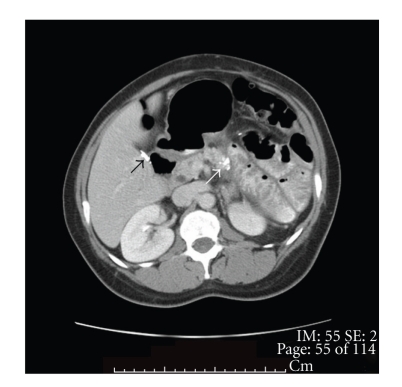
46-year-old woman with pancreatic cancer and prior distal pancreatectomy. CT scan with contrast through the pancreas. There is calcification at the cut surface of the pancreas (white arrow). No residual tumor is seen at this site. Cholecystectomy clips are present in the gall bladder fossa (black arrow).
